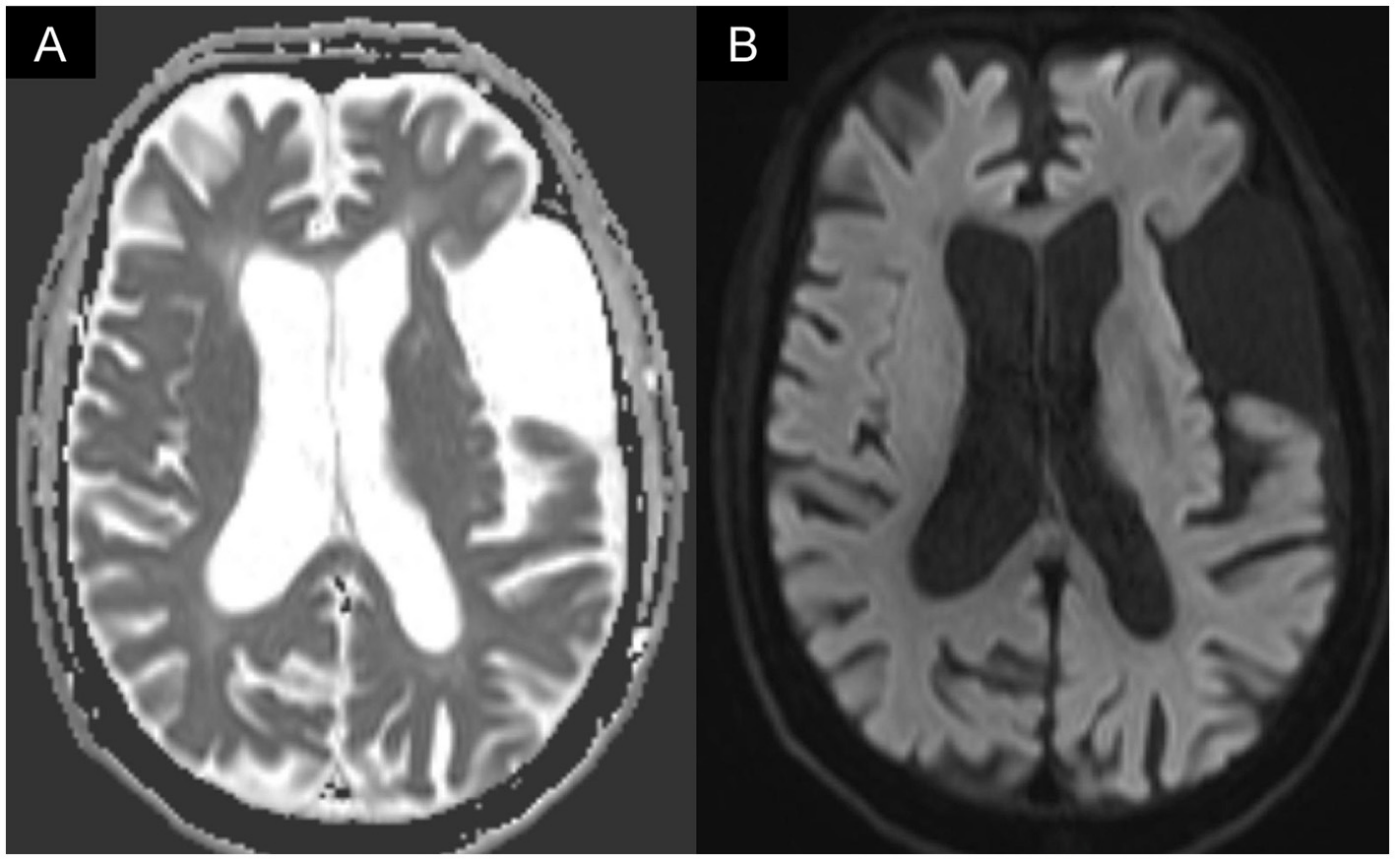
Brain MRI showed (A) increased signal intensity on diffusion-weighted imaging (DWI) in the left temporal lobe, which corresponded to (B) decreased apparent diffusion coefficient (ADC) value. The MRI image was compatible with post-stroke encephalomalacia but found no evidence of new infarction.
